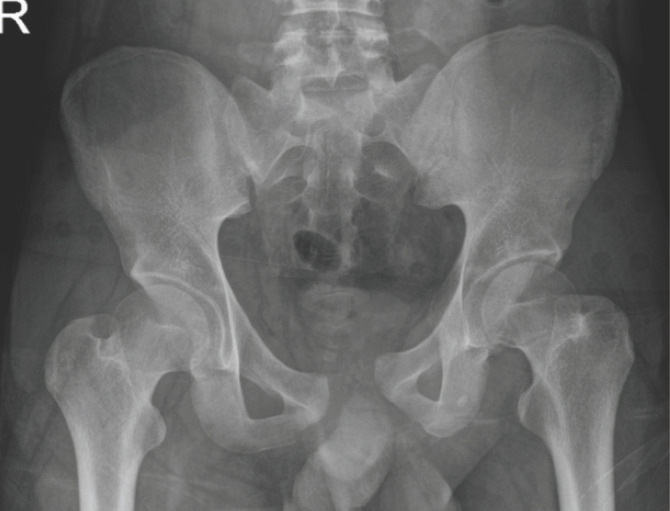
Pre-operative pelvic radiograph.
